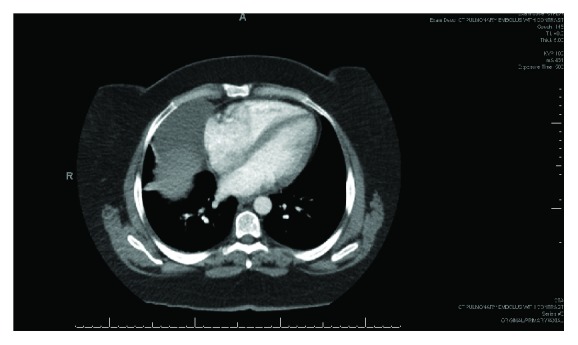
CT chest with contrast demonstrating a pericardial cyst.
